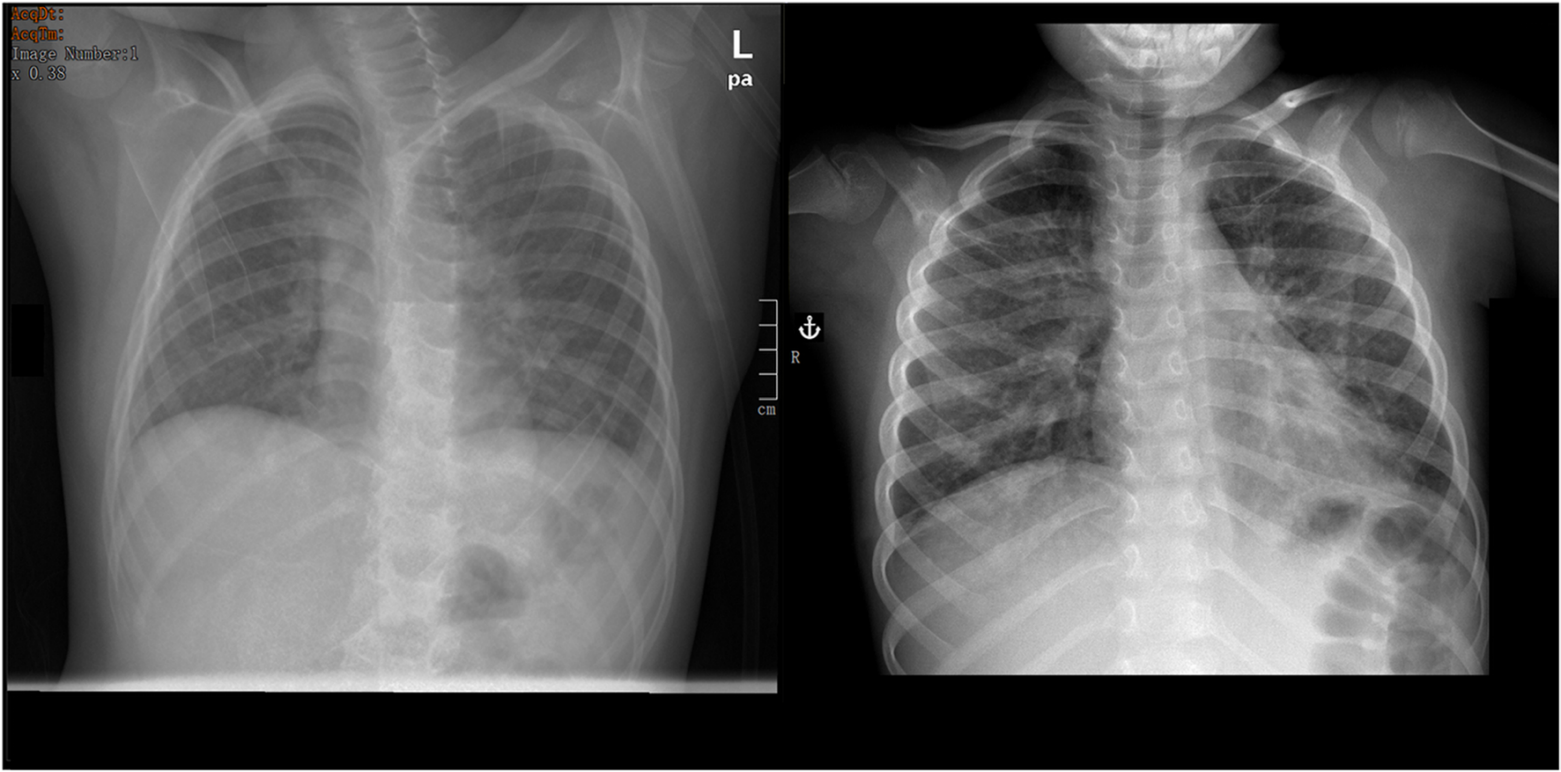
Chest X-ray showing migratory infiltrates indicative of eosinophilic infiltration
